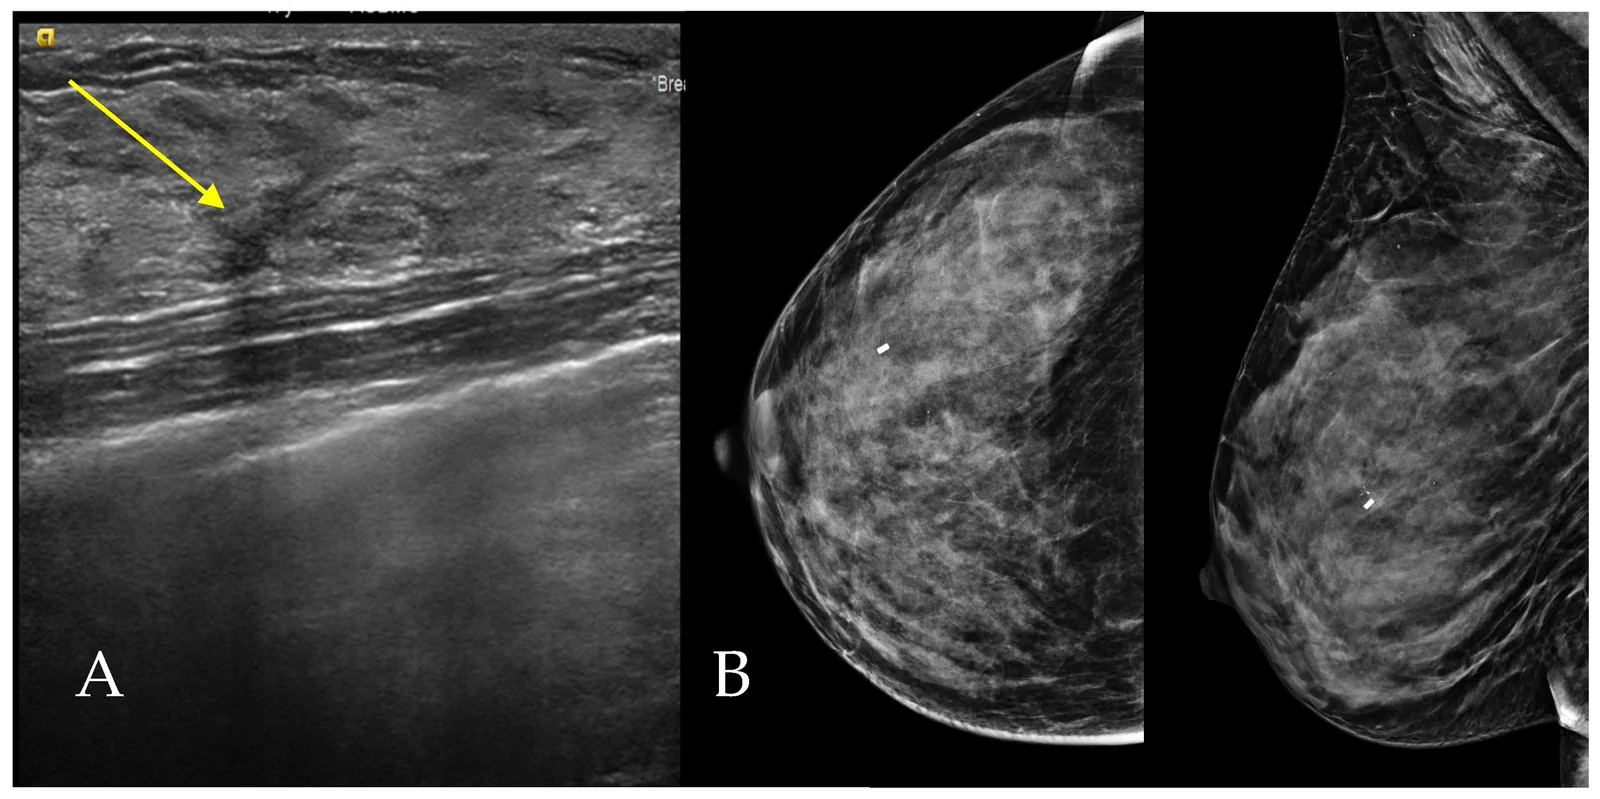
(A) Ultrasound of the right breast showing post-biopsy scarring appearing as a linear hypoechoic non-mass lesion adjacent to the clip marker (arrow). (B) 2D CC and MLO mammography of the same patient during the same visit demonstrating the clip marker but with no mammographically visible post-biopsy scarring.
